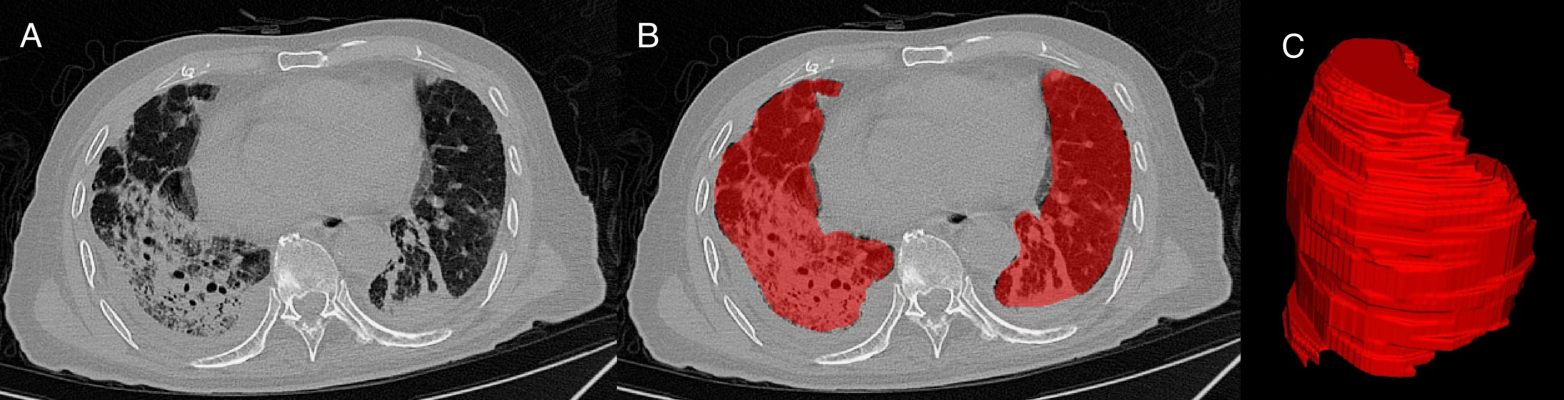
CT-based lung lesion segmentation and 3D reconstruction. (A) Original CT image. (B) Manual segmentation of the region of interest (ROI) on CT images (highlighted in red). (C) Three-dimensional reconstruction of the ROI.
